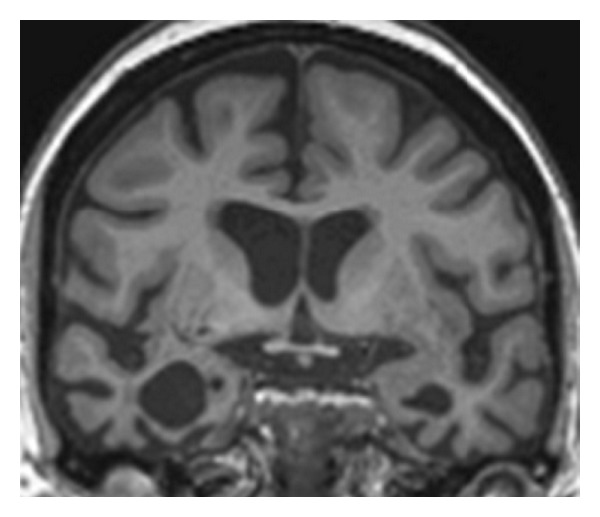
Representative coronal slice through the anterior temporal lobes from brain MRI in LC, six years after onset of symptoms (the right hemisphere is displayed on the left). There is selective atrophy of the anteroinferior and mesial temporal lobes including amygdalae and hippocampi (more marked on the right) and less marked atrophy of perisylvian cortices bilaterally.
